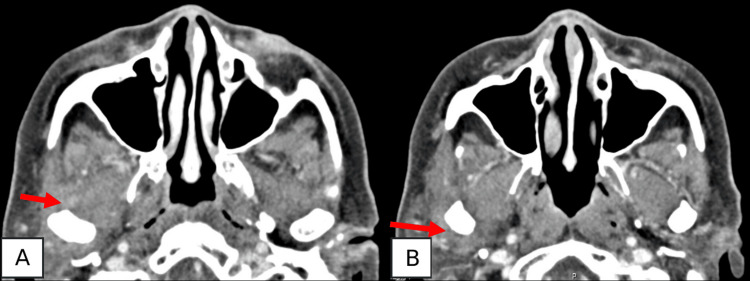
Computed tomography scan two weeks after arthrocentesis Complete resolution of the abscess collections (red arrows), anteriorly (A) and posteriorly (B)
